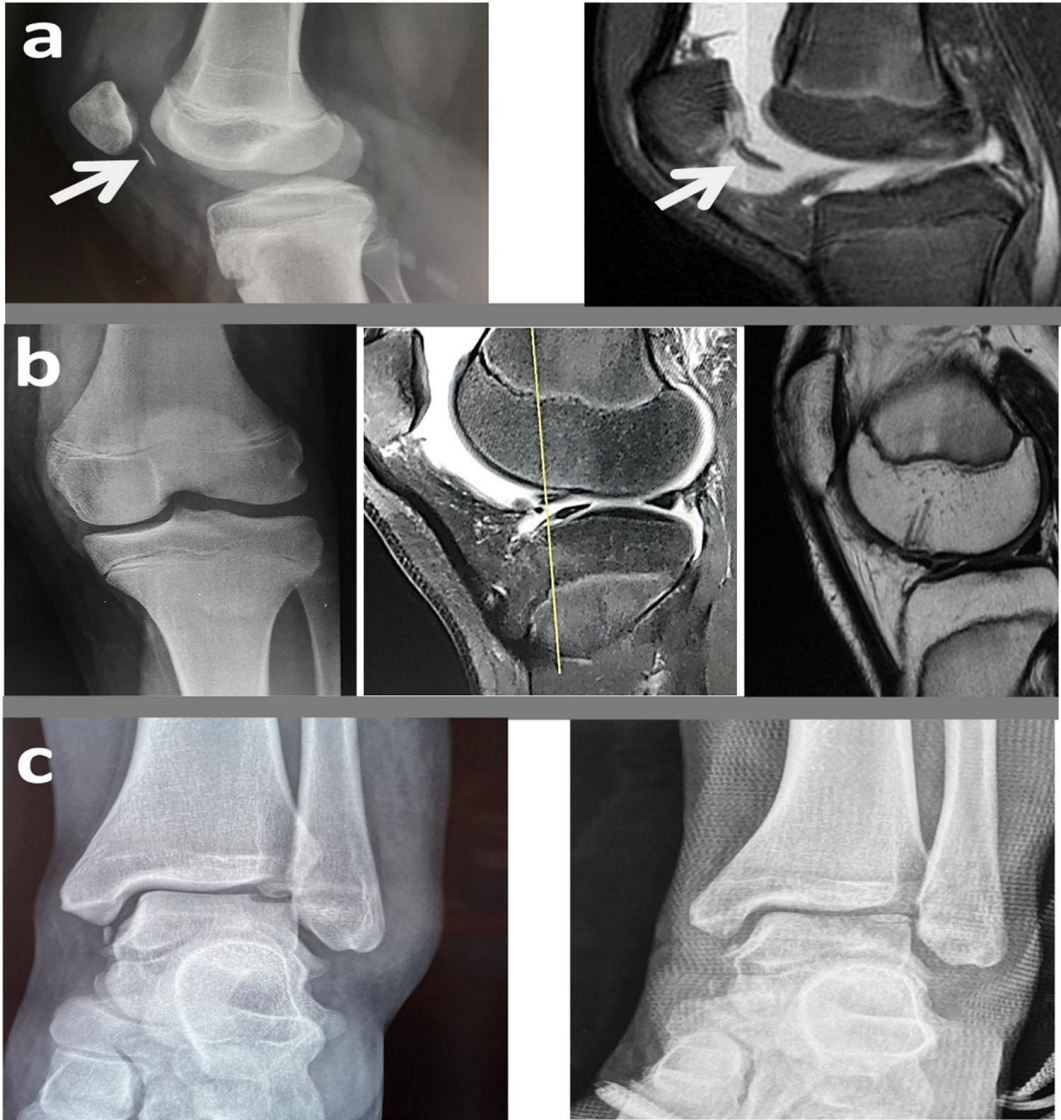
Imaging pre- and postoperatively for the 3 types of management: a free fragment in the knee treated by mini open fixation (preoperative plain radiograph and MRI); b knee osteochondral lesion treated by arthroscopic fixation (preoperative plain radiograph, preoperative MRI, and postoperative MRI); c talus-free fragment treated by mini open fixation (pre- and postoperative plain radiograph)
